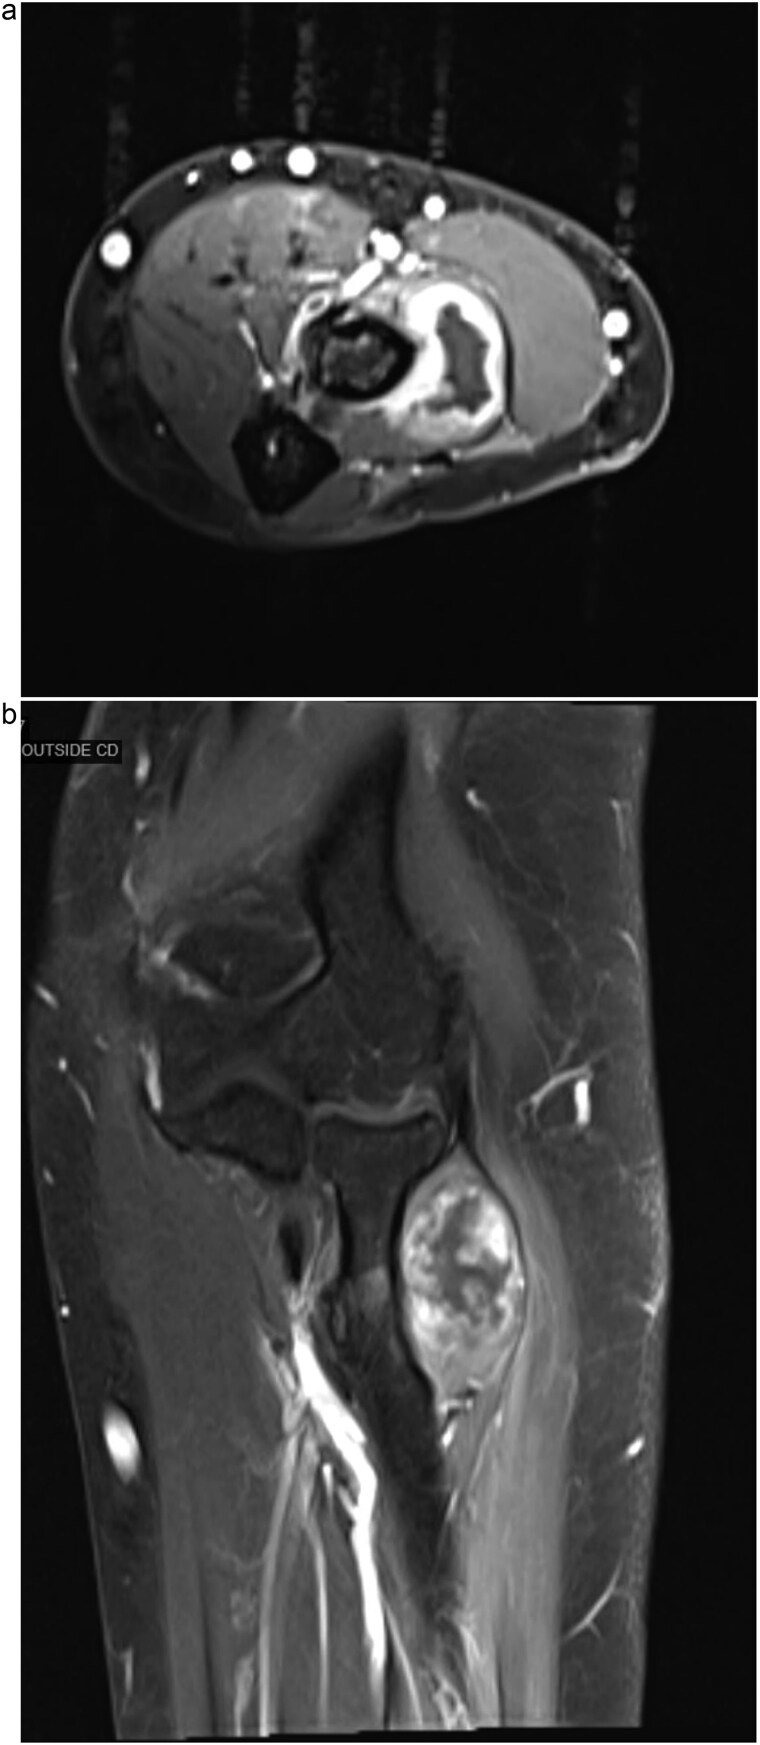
(a) Axial MRI of the forearm demonstrating a mass within the supinator muscle, associated with compression of the PIN. (b) Coronal MRI of the forearm showing a well-defined intramuscular mass within the supinator muscle, with surrounding edema and displacement of adjacent neurovascular structures.
